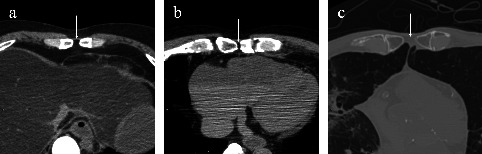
(a) Computed tomography image of a case of adipose tissue present immediately below the sternal foramen (indicated by the arrow). The pericardium can be seen in (b), and the lungs in (c). These are postmortem images of different bodies.
